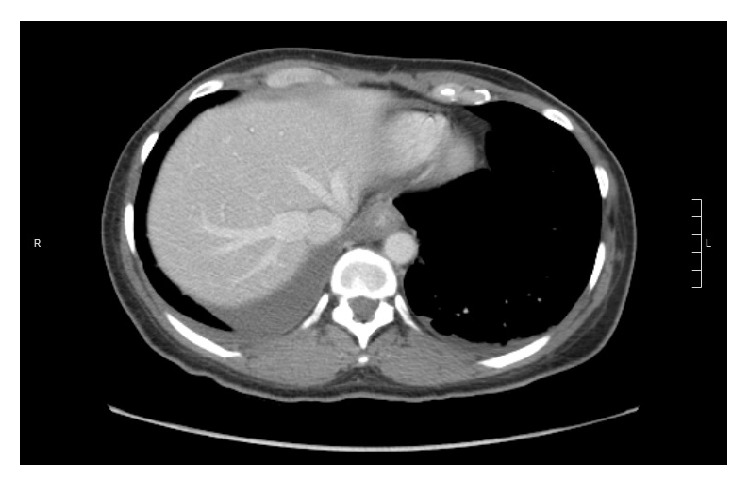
Abdominal CT scan revealing thickening of the distal esophagus.
